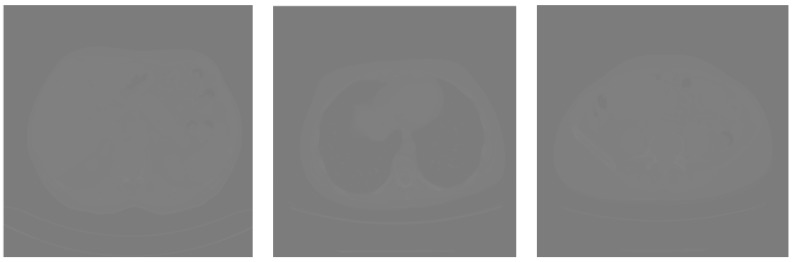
Raw CT slices in DICOM format for three different patients imported from the IRCAD dataset [50].
